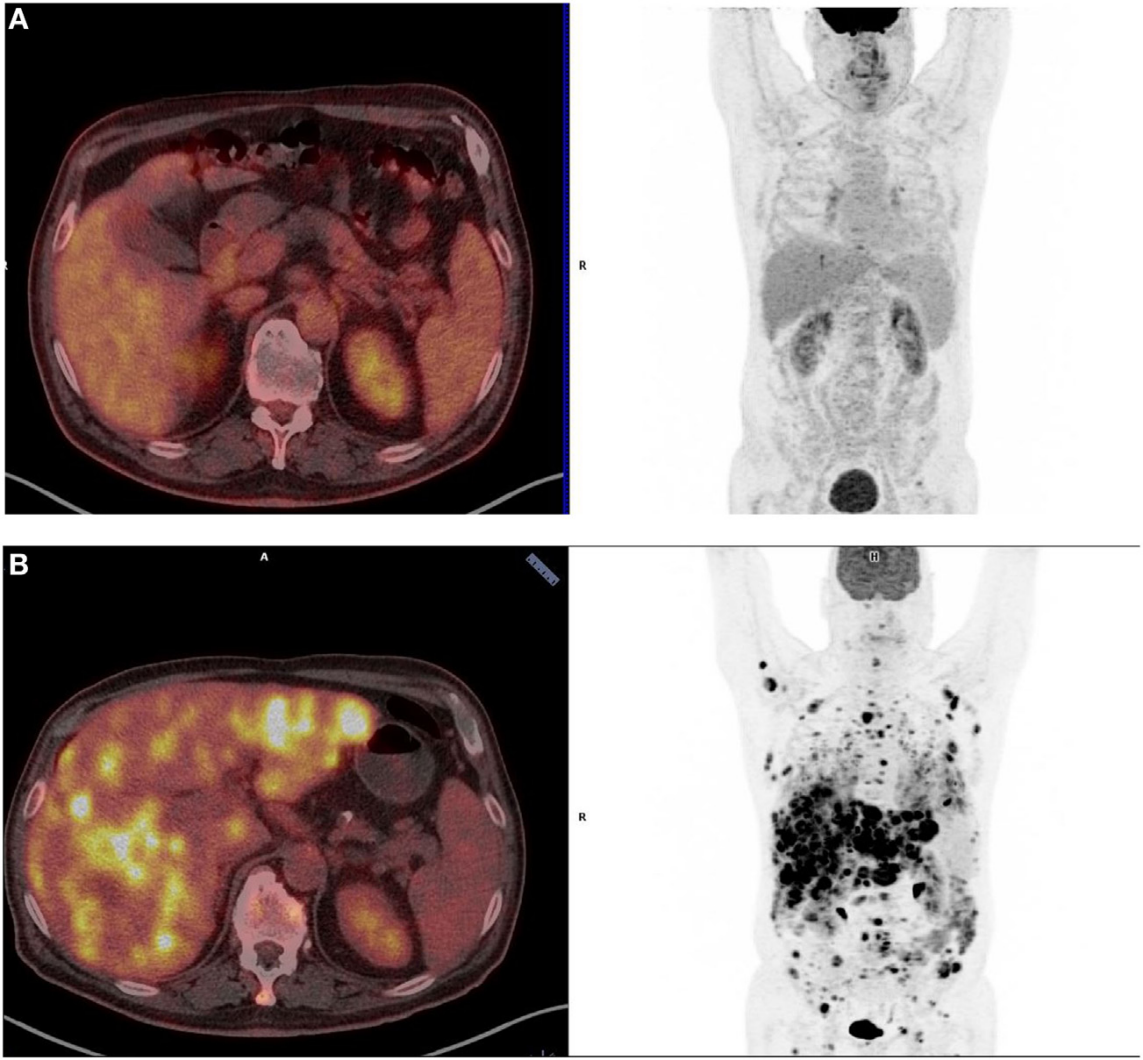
Positron-emission tomography (PET)-scanner imaging at baseline (A) and after three cycles of pembrolizumab (B). (A) Patient’s baseline PET scan (December 2016): only four infracentimetric hypermetabolic lung nodules are visible [standardized uptake values (SUV) from 2.2 to 5.5]. No evidence of distant metastases elsewhere. (B) Patient’s PET scan after three cycles of pembrolizumab (May 2017): diffuse and multiple hypermetabolic lung, liver, subcutaneous tissue, and peritoneal lesions (SUV from 3.5 to 17).
